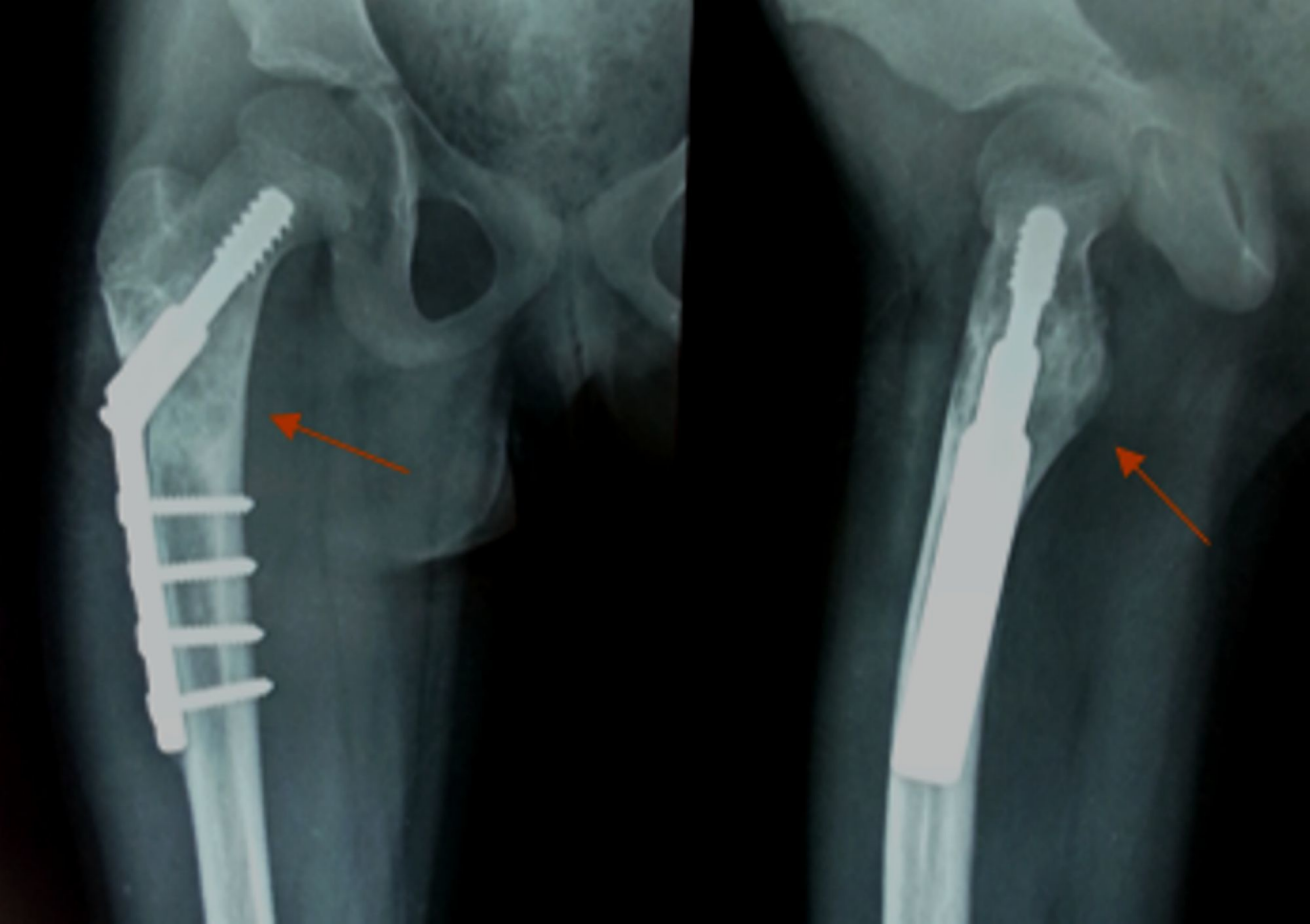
Final follow-up radiographs at 19 months Shows complete healing of the lesion and incorporation of the bone graft.
